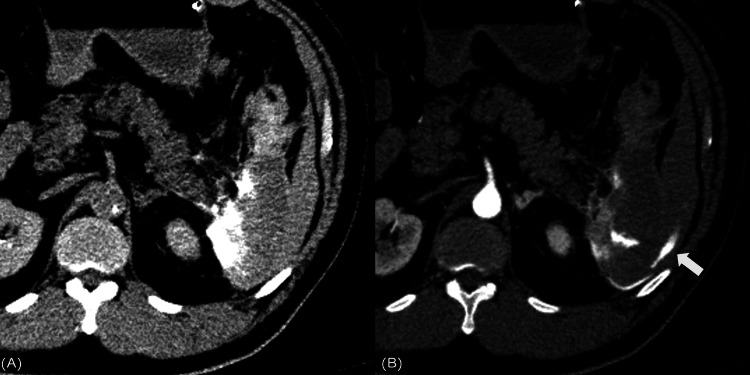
Second CT examination (A) Non contrast-enhanced CT after the first embolization indicating a peri-splenic contrast accumulation. (B) Subsequent contrast-enhanced CT arterial phase indicating extravasation (arrow). CT, computed tomography
